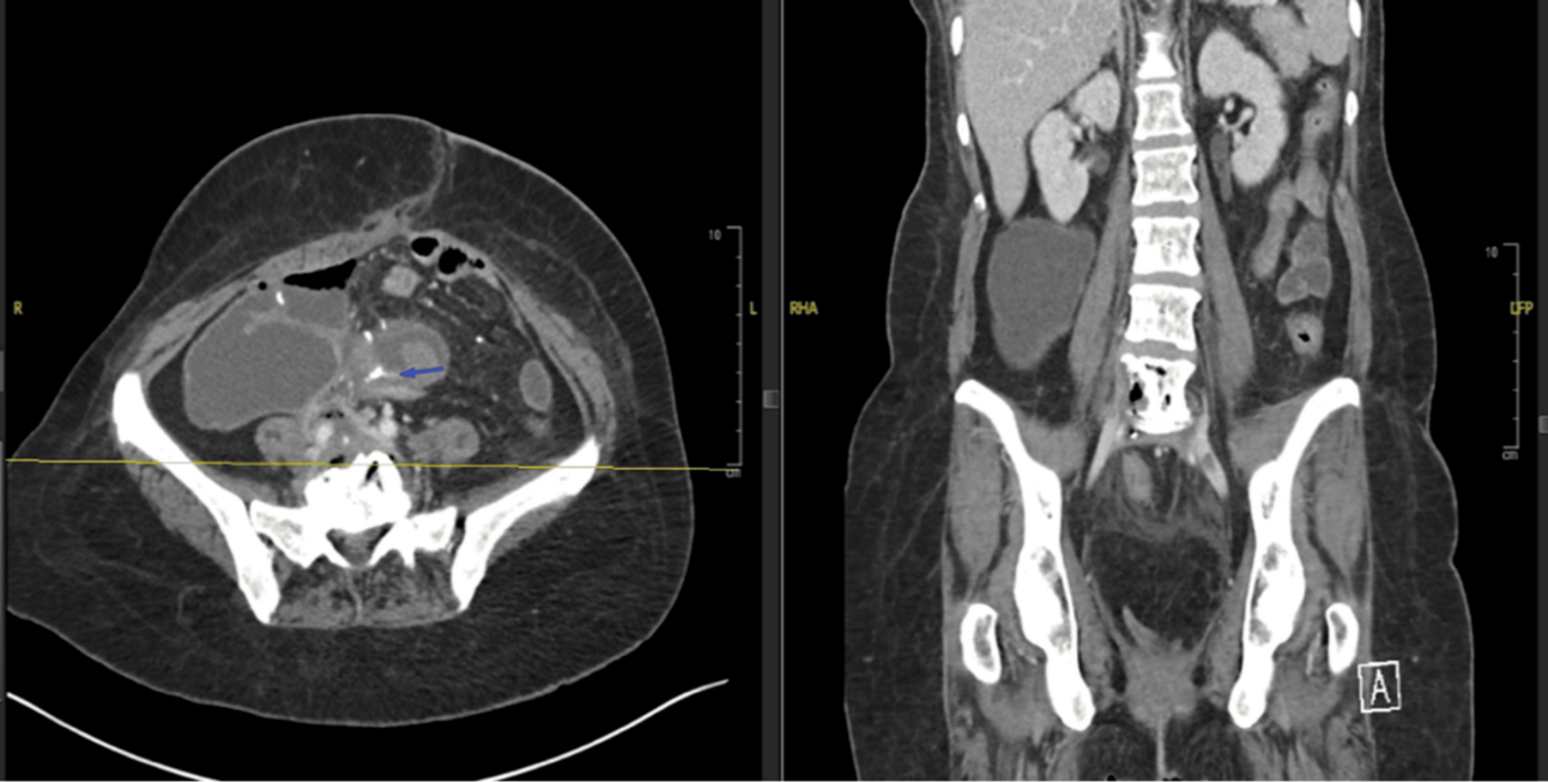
CT hyperdense signal pointed by the blue arrow indicated the sigmoidal fistula (Left). Cortical erosion was along the anterior surface of the L5 vertebral body (Right).
